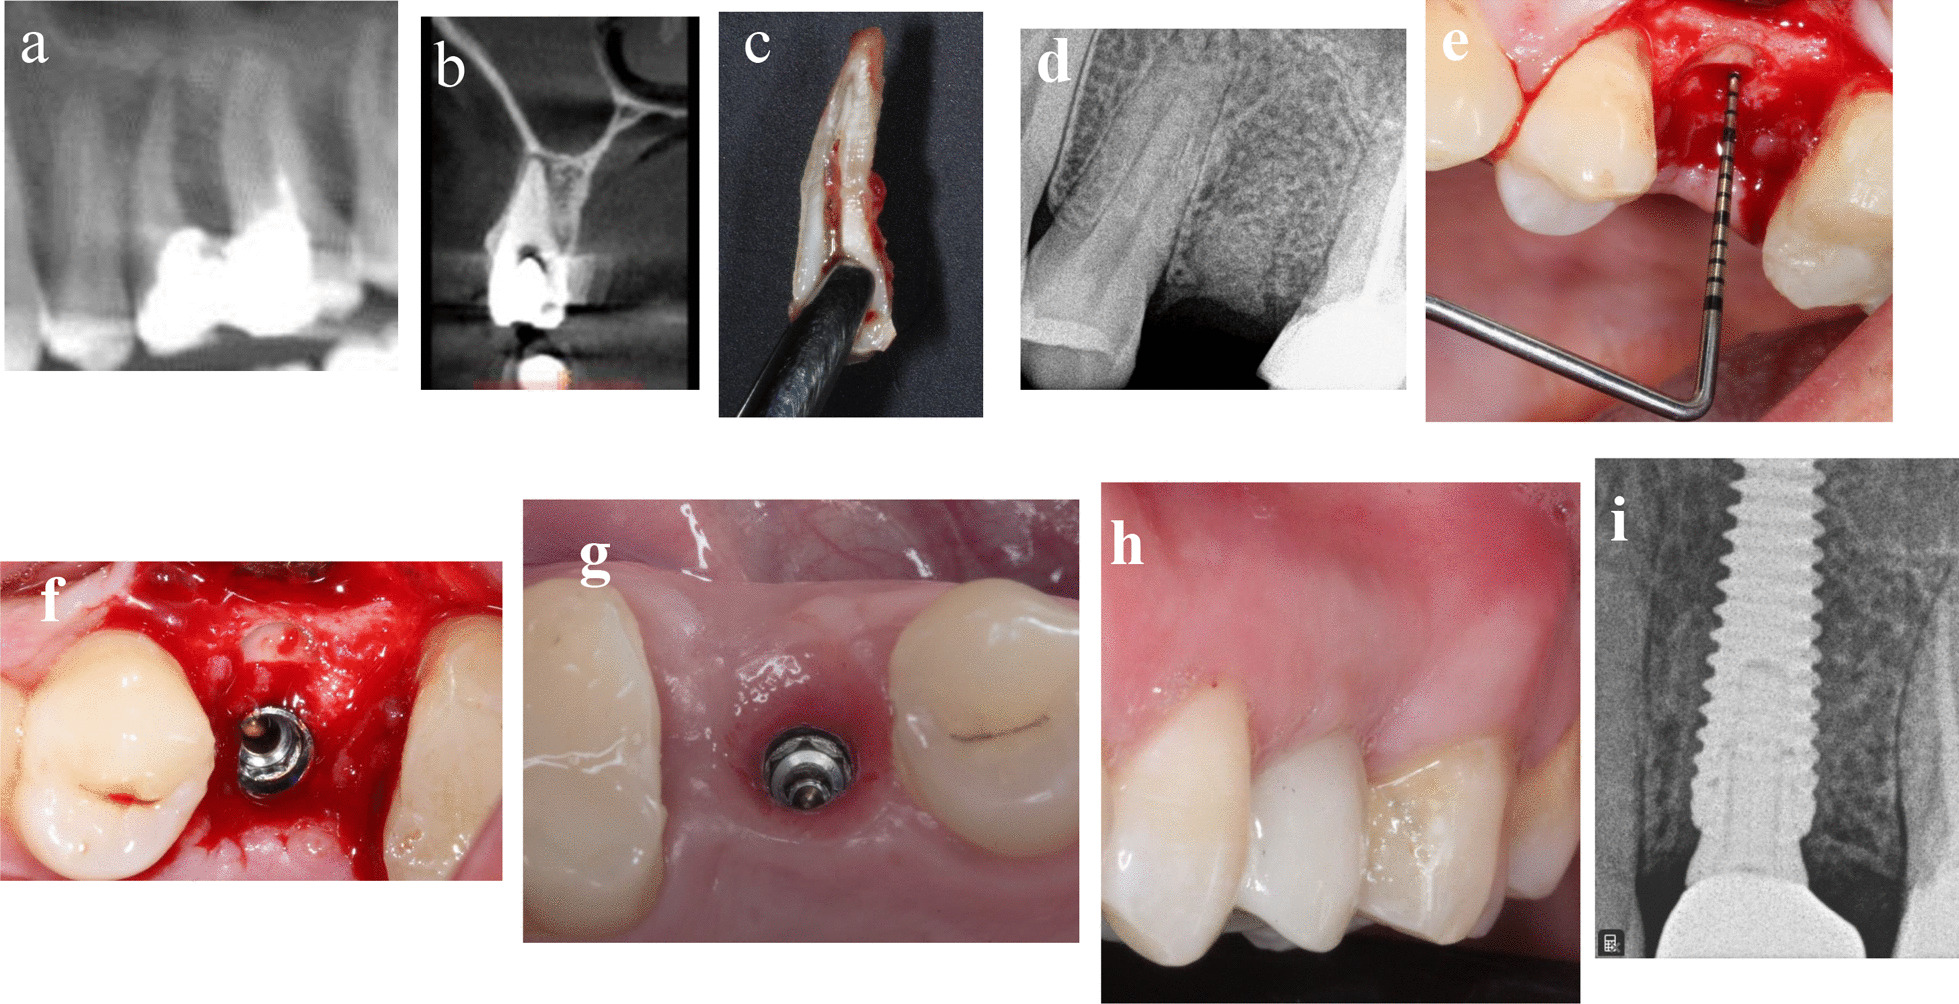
a–i Photos and radiographs of case #1 replacing maxillary left second premolar. a Panoramic view of preoperative CBCT; b cross-sectional view of preoperative CBCT; c the extracted palatal portion with the apex; d periapical radiograph immediately after shield preparation and bone grafting; e the depth of soft tissue penetration measured with periodontal probe; f implant placed palatal to the shield in healed socket; g occlusal view of the healed peri-implant soft tissue; h frontal view of the final implant restoration at 15 months post-loading; i periapical radiograph at 15 months post-loading
